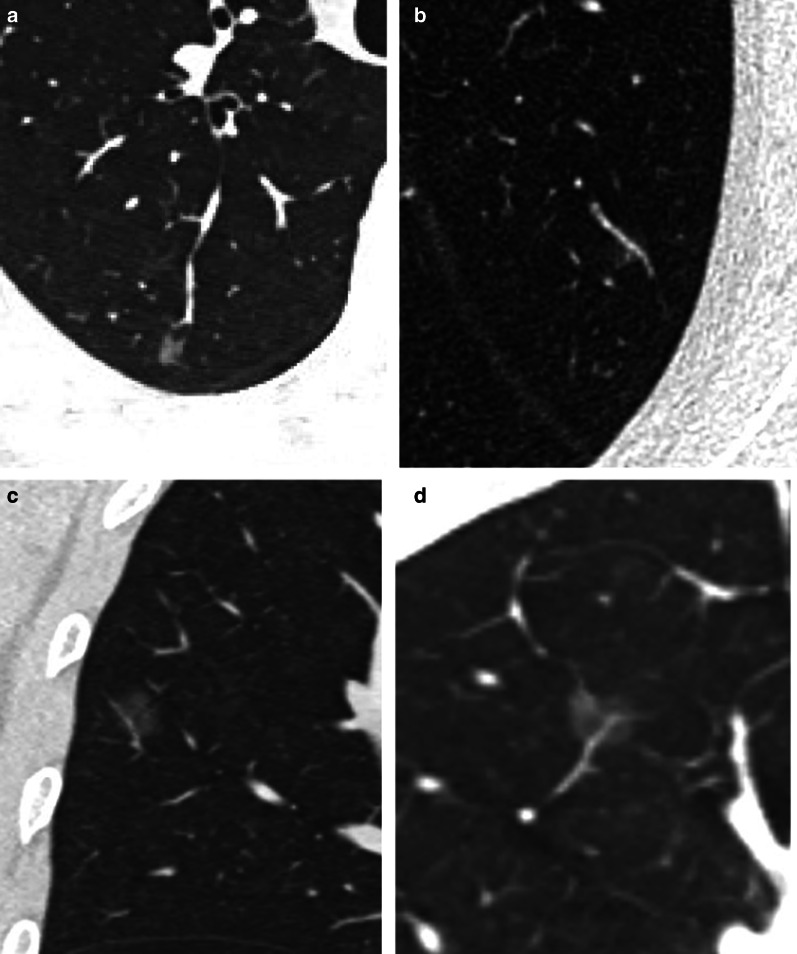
Computed tomography (CT) images of patients with benign ground-glass nodules (GGNs). A 5-mm GGN in the right upper lobe without internal vessels (a). A pulmonary vein (PV) branch without changes is detected in a 6-mm GGN in the left upper lobe (b) and a 9-mm GGN in the right upper lobe (c). A slightly dilated pulmonary artery (PA) branch is detected in a 9-mm GGN in the right upper lobe (d)
